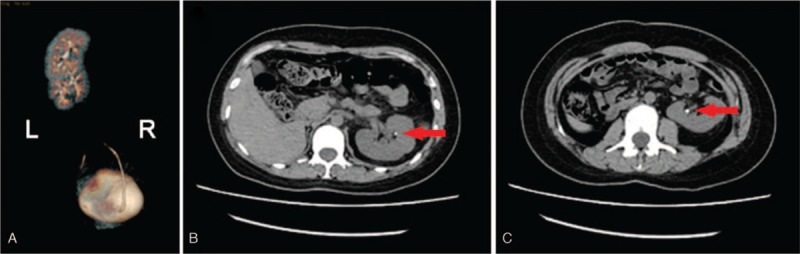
3D CT images of patient 2. (A) 3D CT image revealed lump right-to-left crossed-fused renal ectopia. (B, C) CT demonstrated two 3-mm stones in both renal pelvises.
